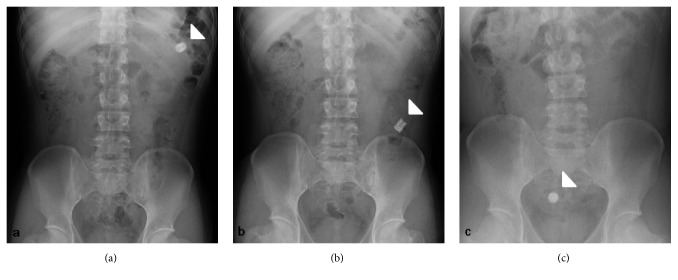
Abdominal radiographs taken at (a) 31, (b) 56, and (c) 218 hours after ingestion showing the patency capsule to be moving anally, but finally becoming retained in the right iliac fossa (arrowheads).
